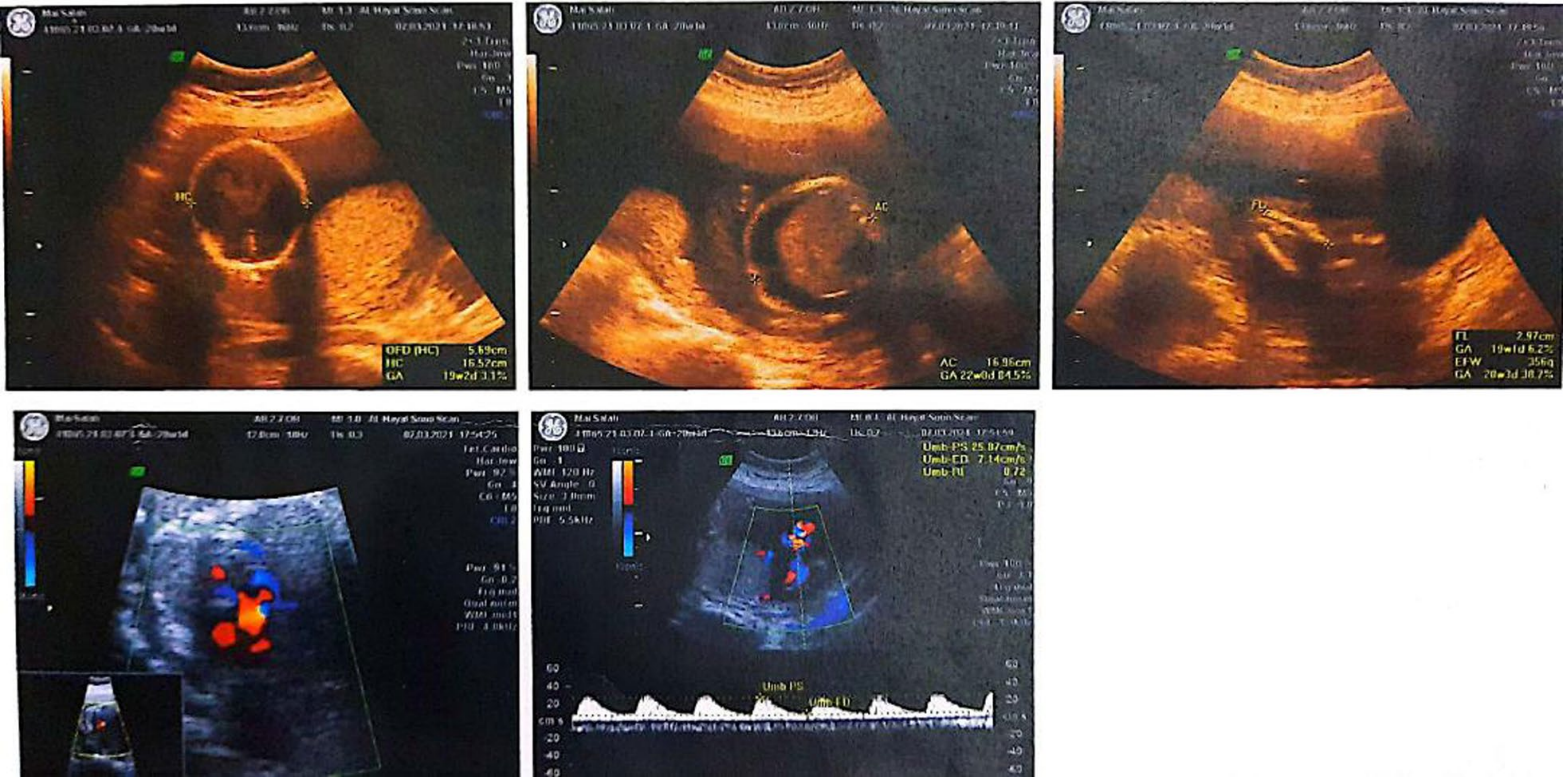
4D obstetric ultrasonography in the 20th gestational week
